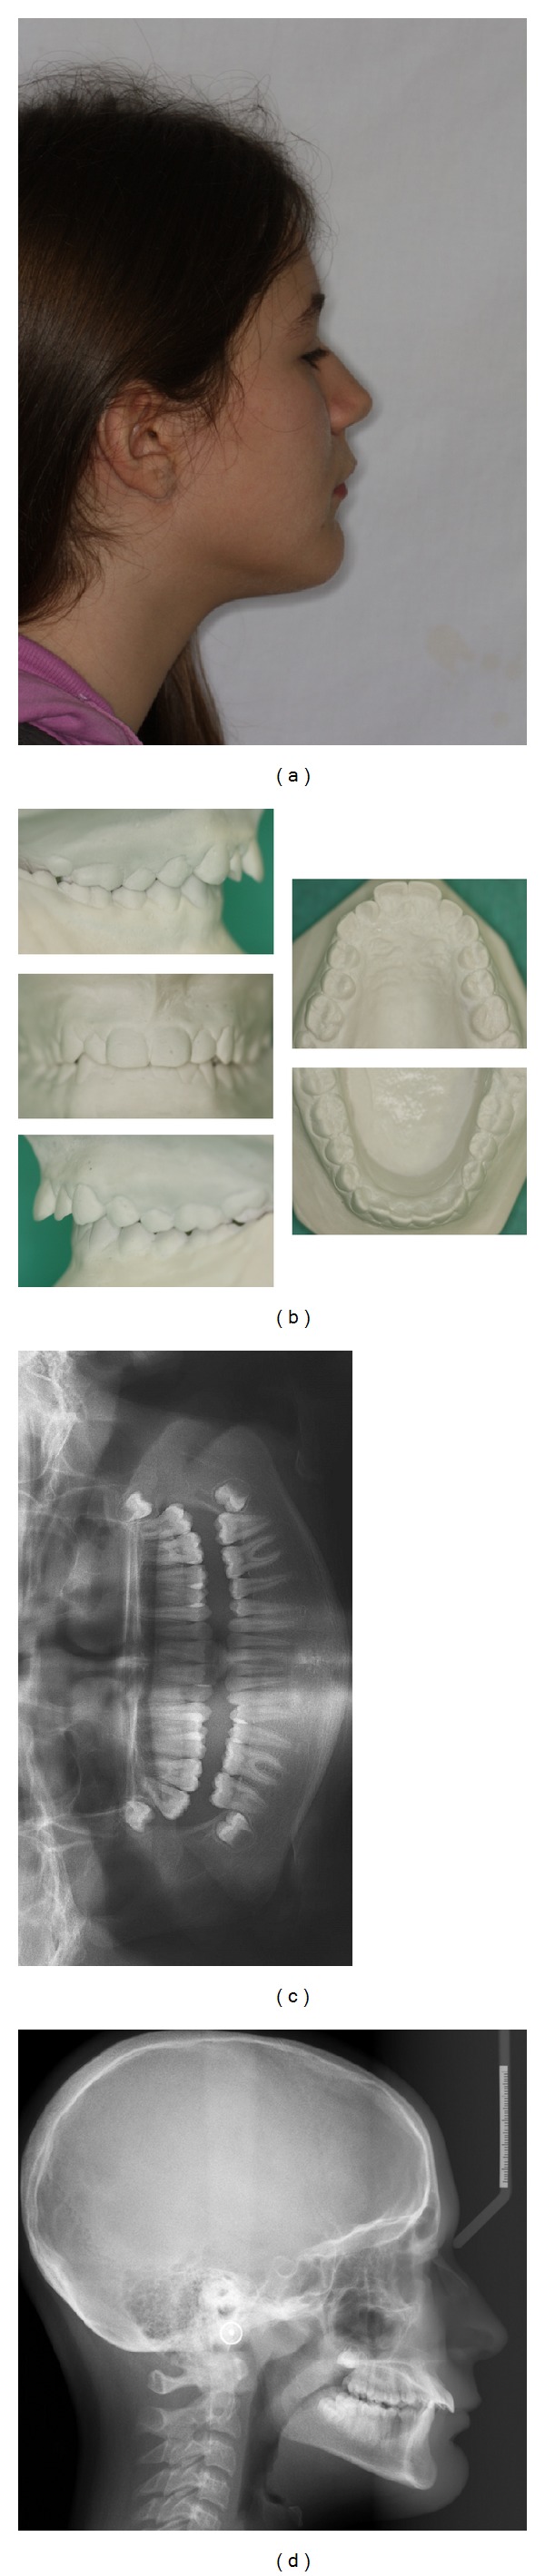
Pretreatment records: (a) extraoral profile photo, (b) dental casts, (c) panoramic radiograph, and (d) cephalometric radiograph.
